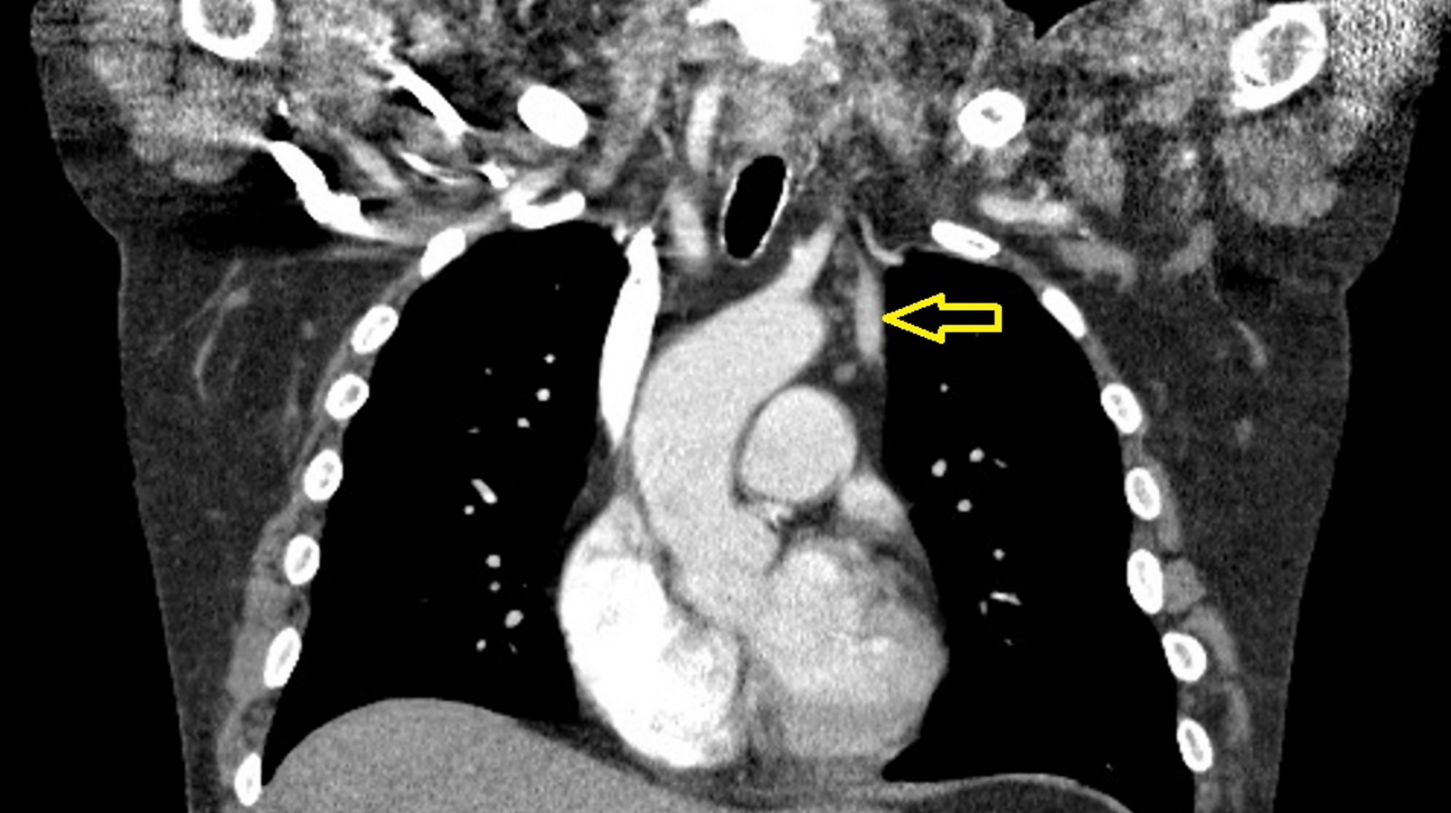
Chest computed tomography (CT) scan (coronal view) with contrast performed on a prior admission confirming the presence of a duplicated superior vena cava (SVC) (marked by yellow arrow) draining into the coronary sinus.
